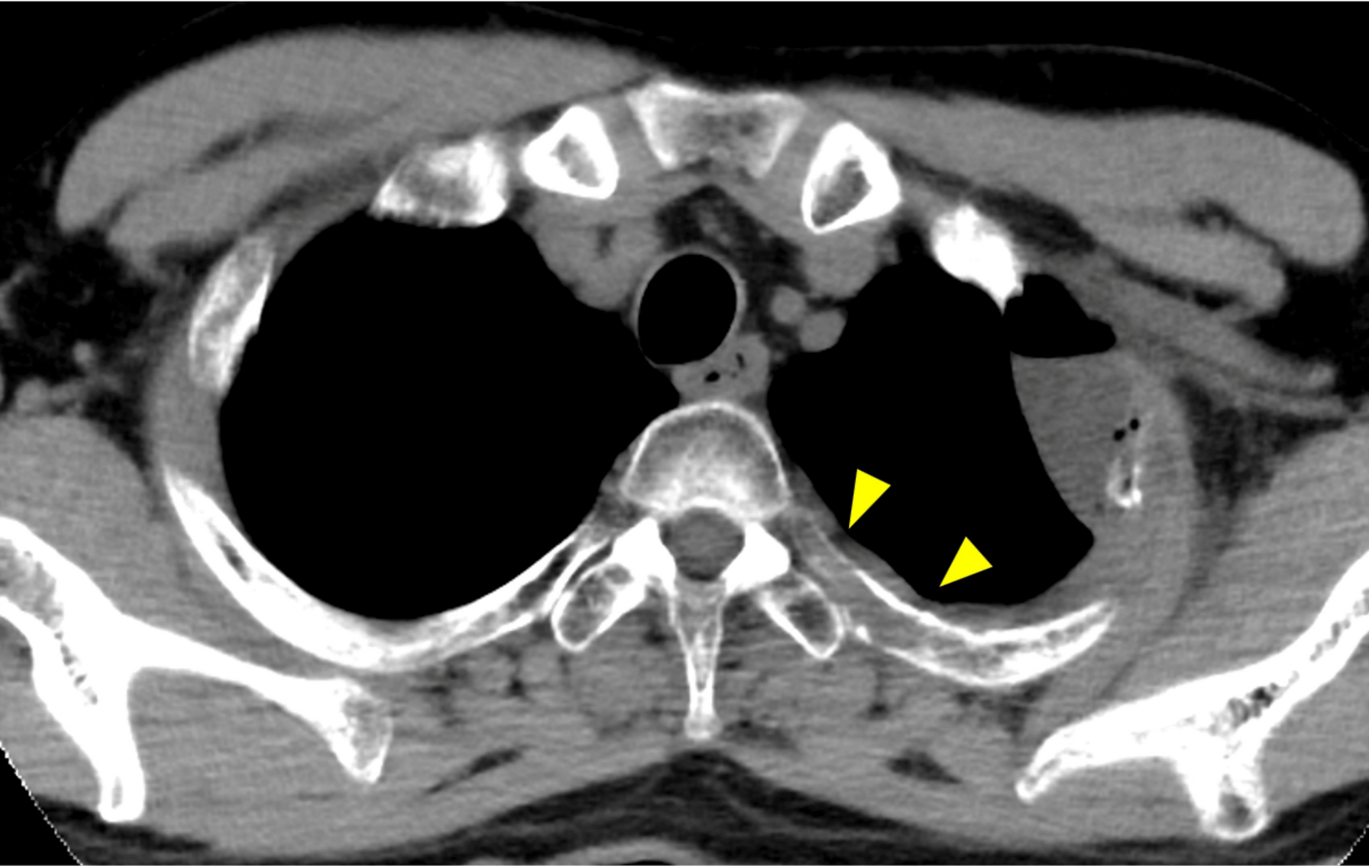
Image findings of rib bone metastasis CT showing the osteolytic lesion in the left third rib (arrow head) in April 2020.
